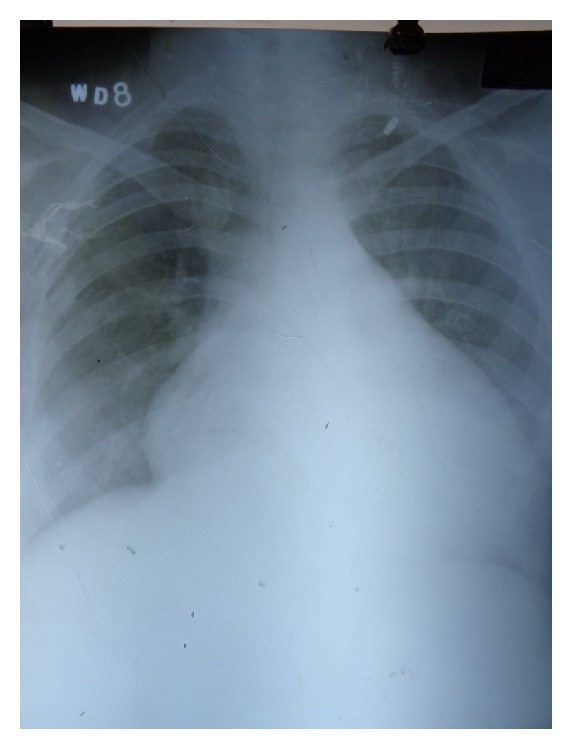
Chest X-ray showing increased cardiothoracic ratio and prominent left pulmonary conus.
